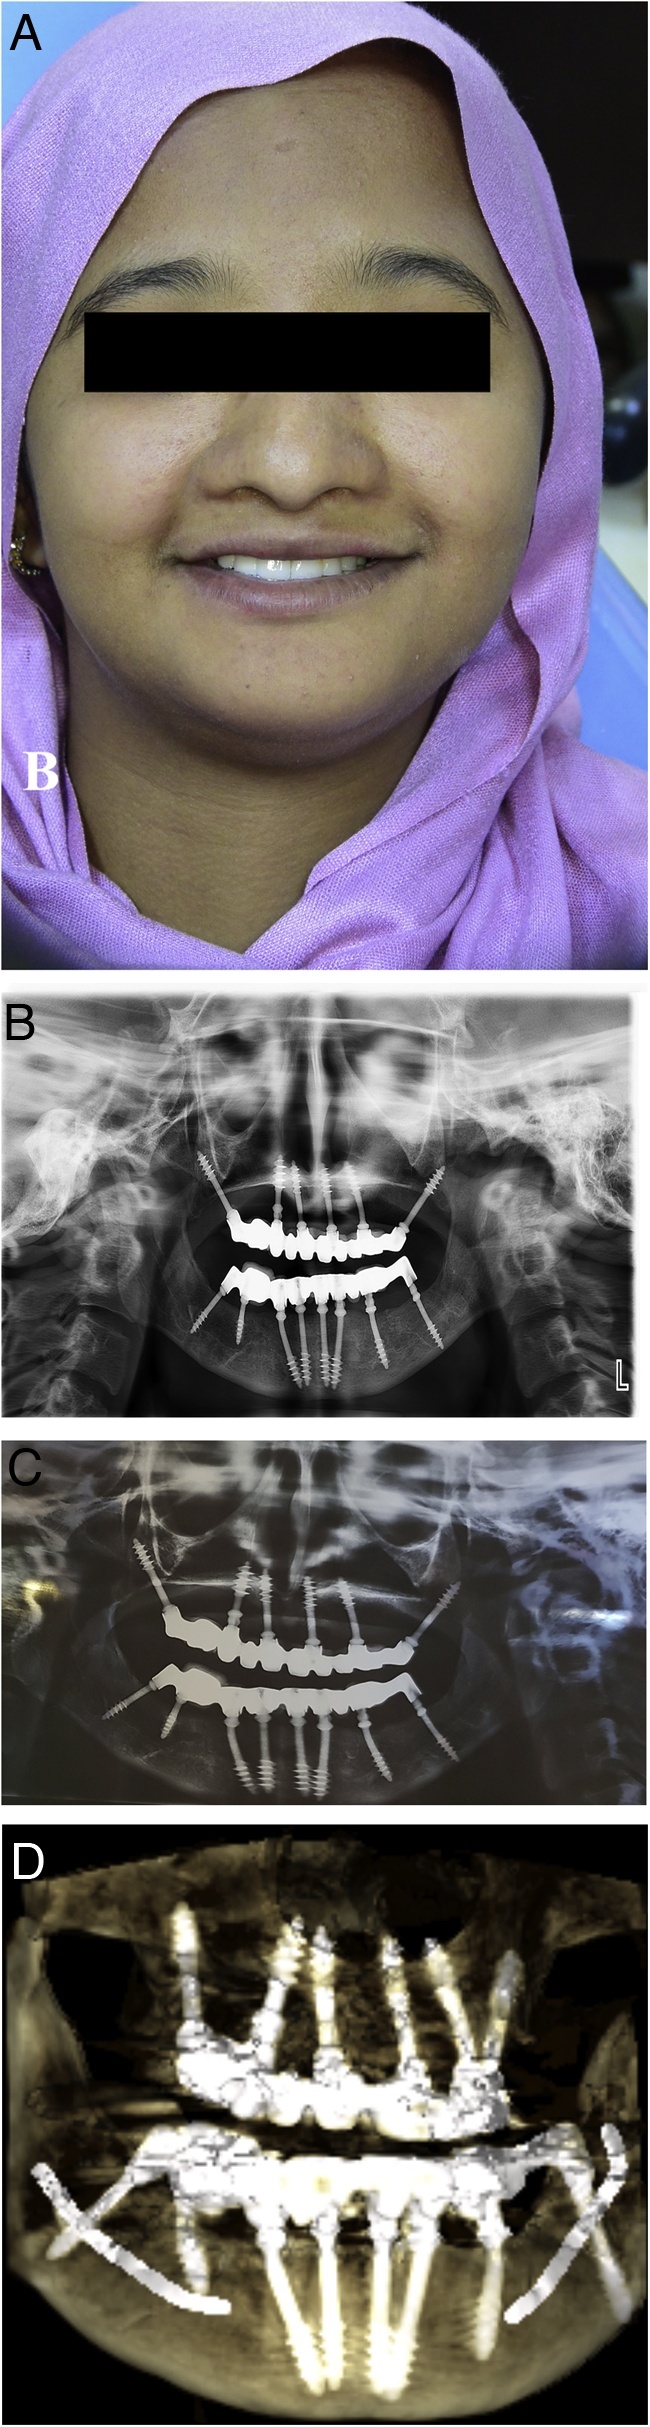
Postoperative and follow-up images of immediately loaded basal implant-supported fixed prostheses fabricated for a 24-year-old woman with cleidocranial dysplasia. A. A frontal view shows the inserted maxillary and mandibular basal-implant supported prostheses. B. A panoramic radiograph shows the maxillary and mandibular prostheses. C. A panoramic radiograph shows the maxillary and mandibular prostheses after 3 years of function. D. A three-dimensional cone beam computed tomography image shows the maxillary and mandibular prostheses after 3 years of function.
